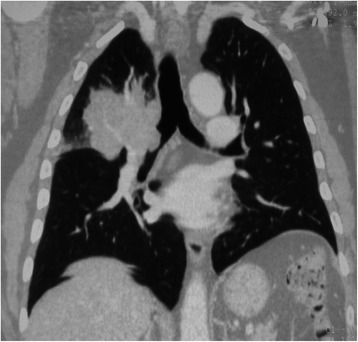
Chest Computed Tomography (CT). Chest CT scan coronal view showing 5-cm right hilar mass bulging into the main bronchus
